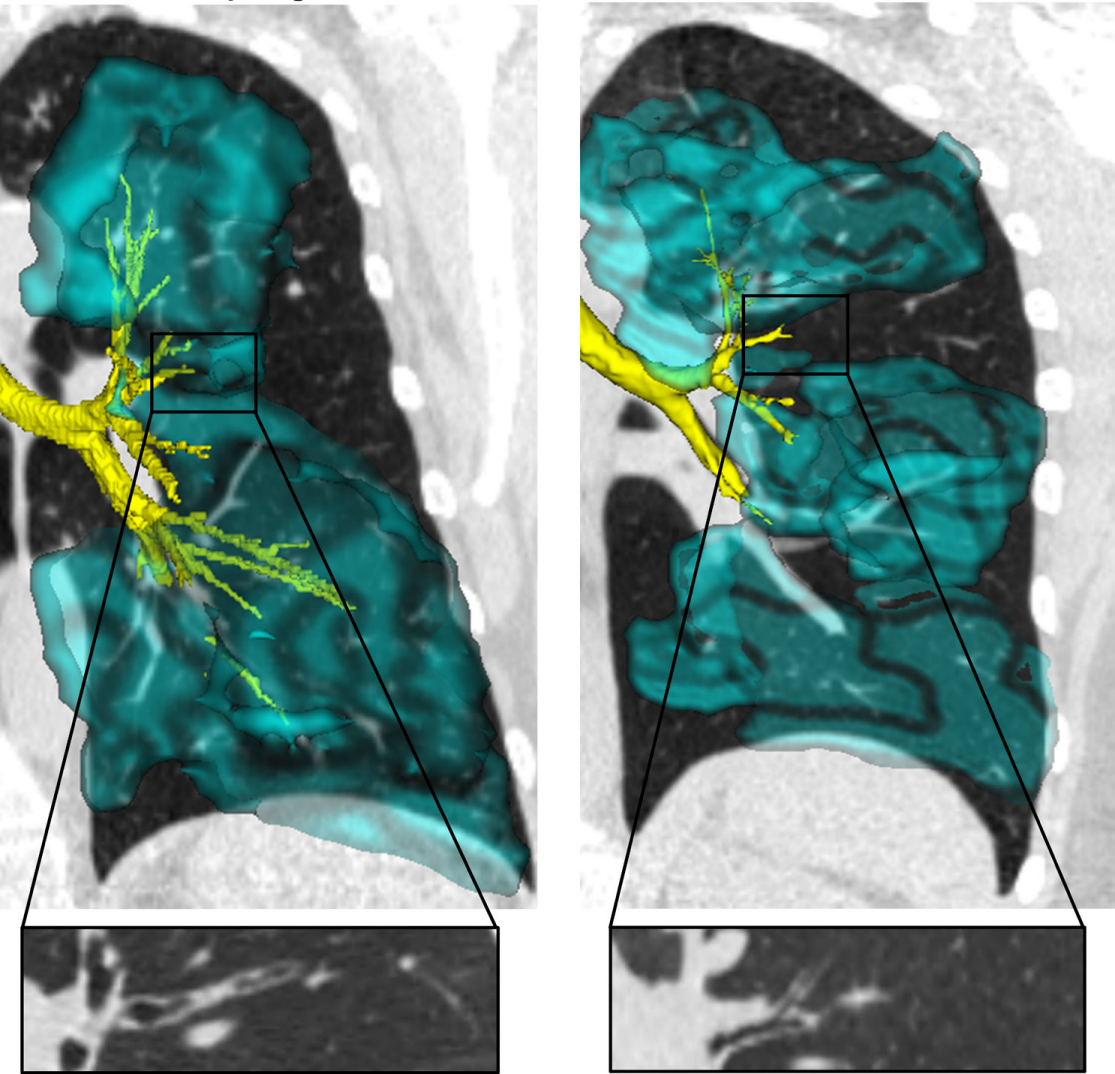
MRI ventilation deficits and relationship to airway abnormalities in two patients with severe asthma. ^129^Xe ventilation (cyan) in three-dimensions co-registered with coronal MDCT reconstructions and airway tree renderings from CT (yellow). The left panels show a left upper lobe posterior ventilation abnormality that was spatially related to an occluded airway shown in the inset with suspected mucus plugging. Right panels show a left lower lobe ventilation abnormality corresponding to airway narrowing (shown in the inset) and numerous ventilation defects distributed throughout the left lung related to airway remodelling and obliteration. Images courtesy of Marrissa J. McIntosh, Harkiran K. Kooner and Grace Parraga, Robarts Research Institute and Department of Medical Biophysics, Western University, London, Canada
